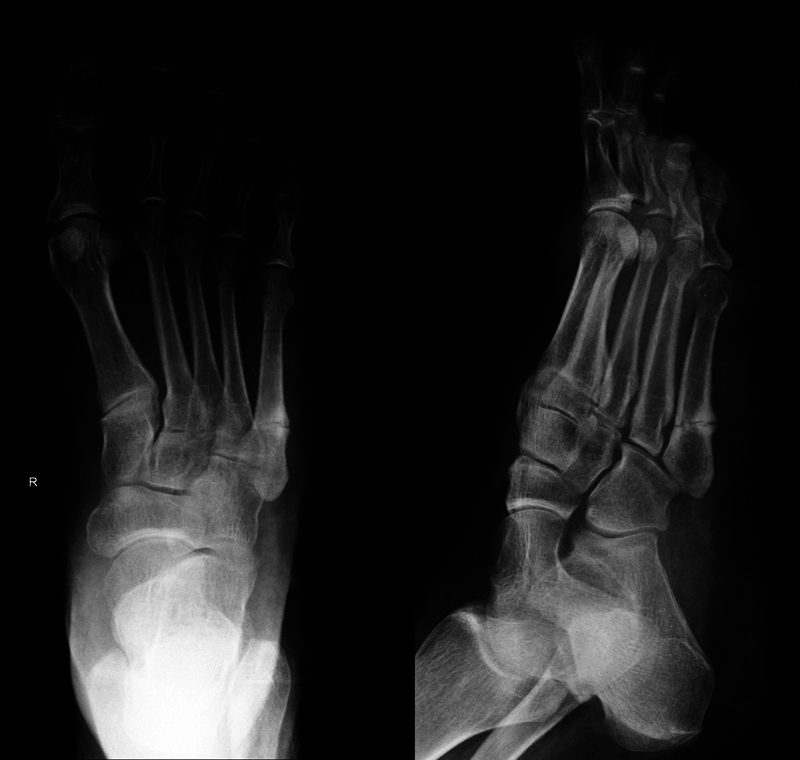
Anteroposterior and oblique radiographs of the right foot.
